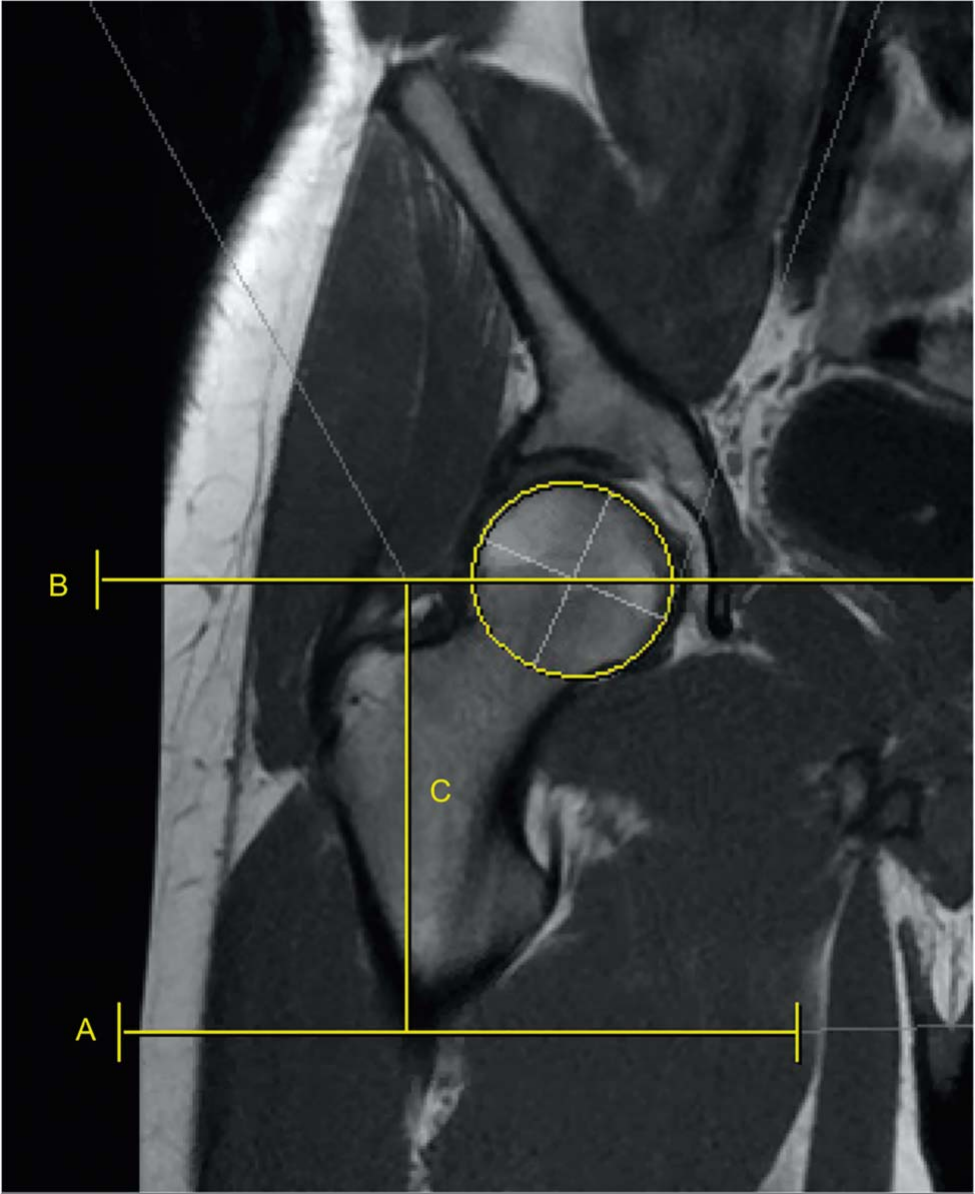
Radiograph showing line A—proximal aspect of gluteal sling (GS), line B—center of femoral head, and line C—measured distance between gluteal sling and center of femoral head.
